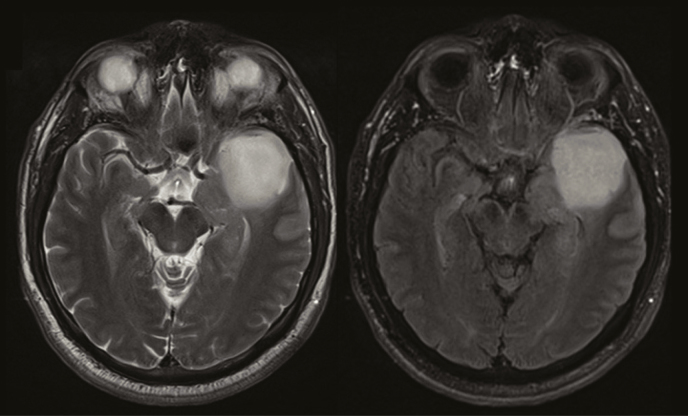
Absence of a T2/FLAIR-mismatch sign in a 36-year-old woman with a left temporal IDH-mutant 1p/19q non-codeleted anaplastic astrocytoma (WHO °III). The tumor demonstrates a near-complete homogeneous hyperintense signal on both T2-w and FLAIR images (right). No peripheral hyperintense rim is visible on FLAIR images.
